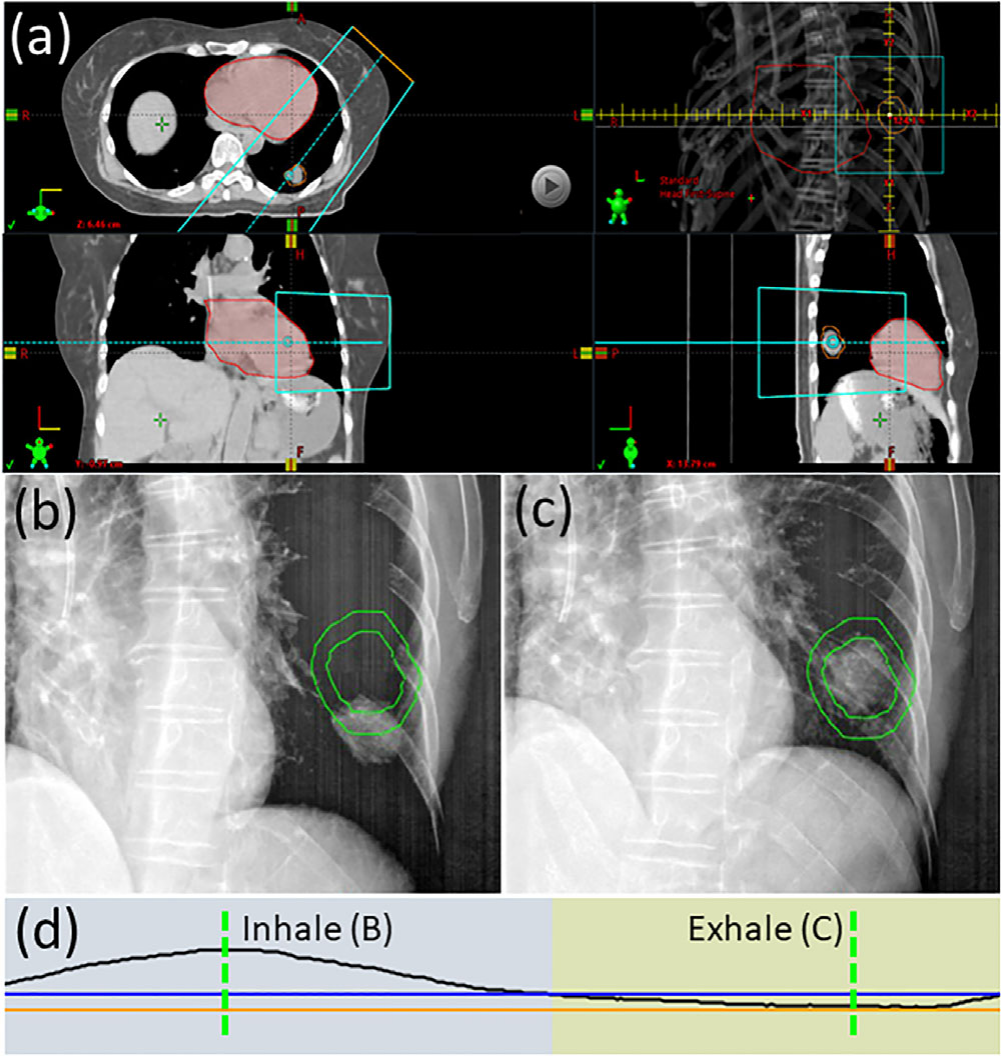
Example of a lung tumor that can be visualized in fluoroscopy with an oblique projection angle. (a) Axial, coronal, and sagittal average CT images, demonstrating that the tumor is positioned posterior to the heart, which will obscure visualization in anterior–posterior projection fluoroscopy. (a) also depicts the beam's eye view of an oblique angled fluoroscopic imaging field, where separation can be observed between the heart (red) and ITV (orange) structures. (b) The ITV and PTV contours (green) and the tumor position on both (b) inhale and (c) exhale oblique fluoroscopy. The corresponding amplitudes of the breathing trace for these images are shown by the dotted green lines in (d). Using fluoroscopy, the gating window (orange and blue lines) is set so that beam‐on (indicated by yellow colorwash) will only occur while the tumor is inside the ITV. This patient is an example of a Gate4060 treatment
